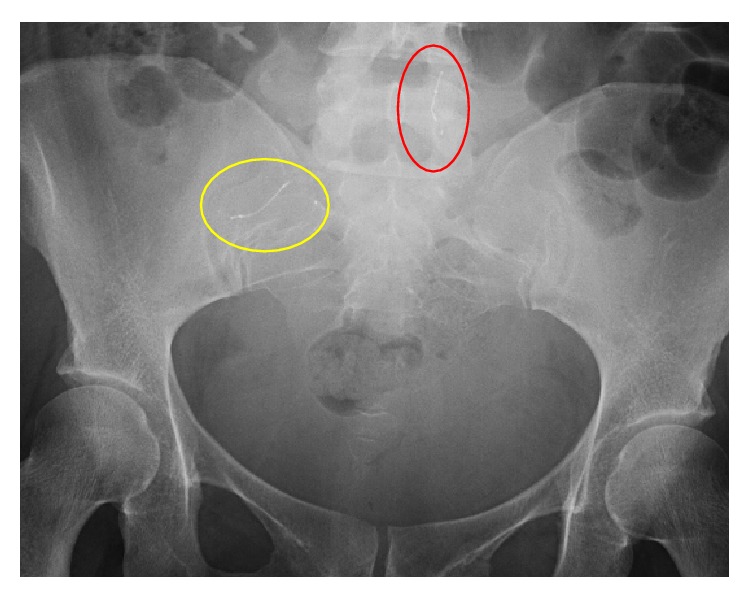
Patient Number 1, postpartum pelvic X-ray 8/30/2014: Essure (circled in red) in the abdomen.
